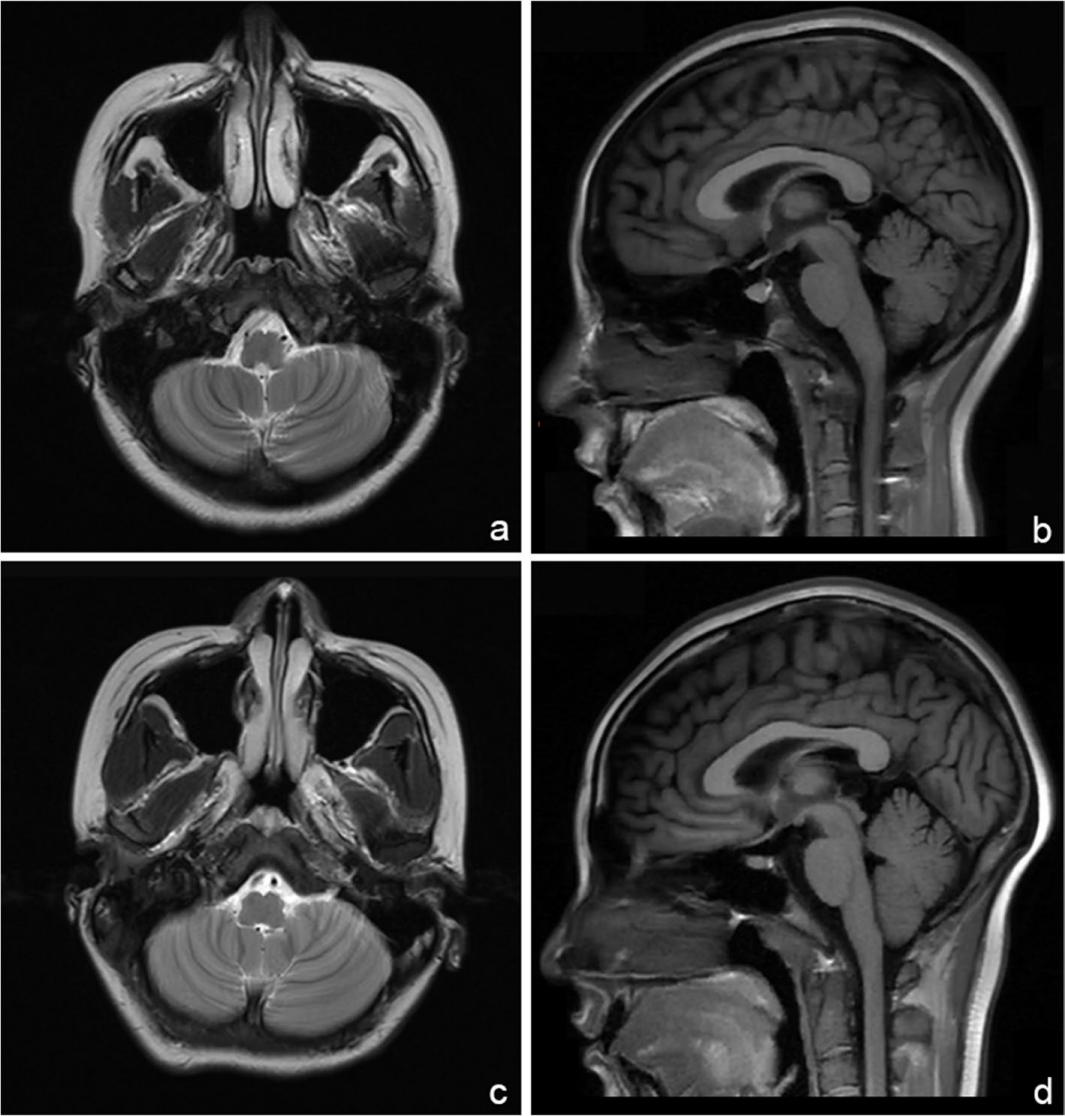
Brain MRI findings of the two patients. Axial T2-weighted imaging and sagittal T1-weighted imaging showed enlarged cerebellar sulci in (a, b) case 1 and (c, d) case 2 relatively, indicating cerebellar atrophies
